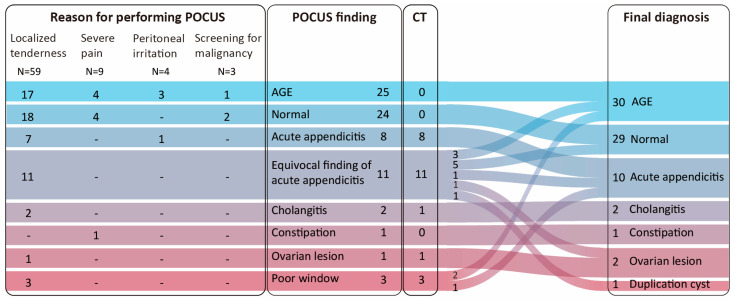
Performance of point-of-care ultrasound in pediatric emergency department. Abbreviations: POCUS, point-of-care ultrasound; CT, computed tomography; AGE, acute gastroenteritis.
